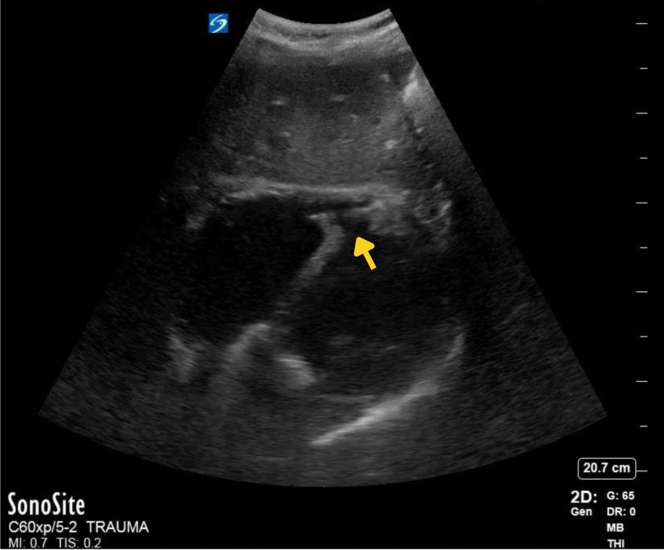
Focused assessment of sonography in trauma (FAST) was performed on a patient who presented after blunt chest wall trauma and did not show any intraperitoneal or pericardial free fluid. However, the subxiphoid FAST view showed a ventricular septal defect (yellow arrow).
